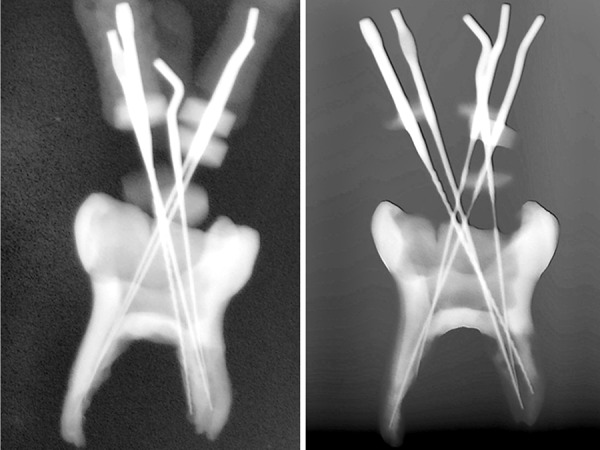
Radiographic view of working length with 5 root canals *in vitro*
